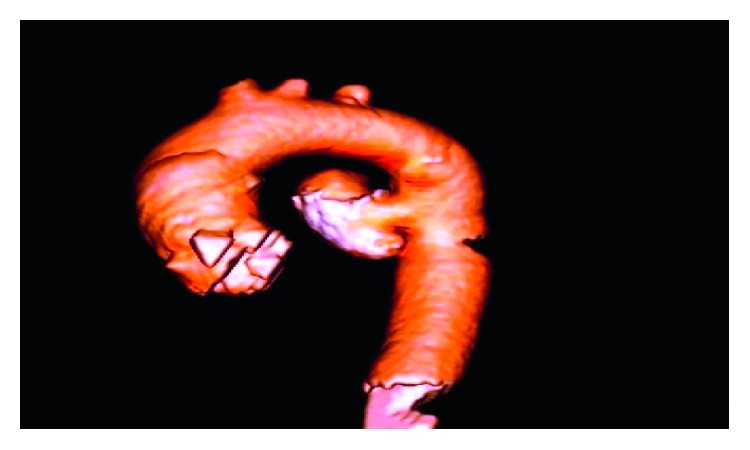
CT 3D shows aneurysm formation in descending aorta.
